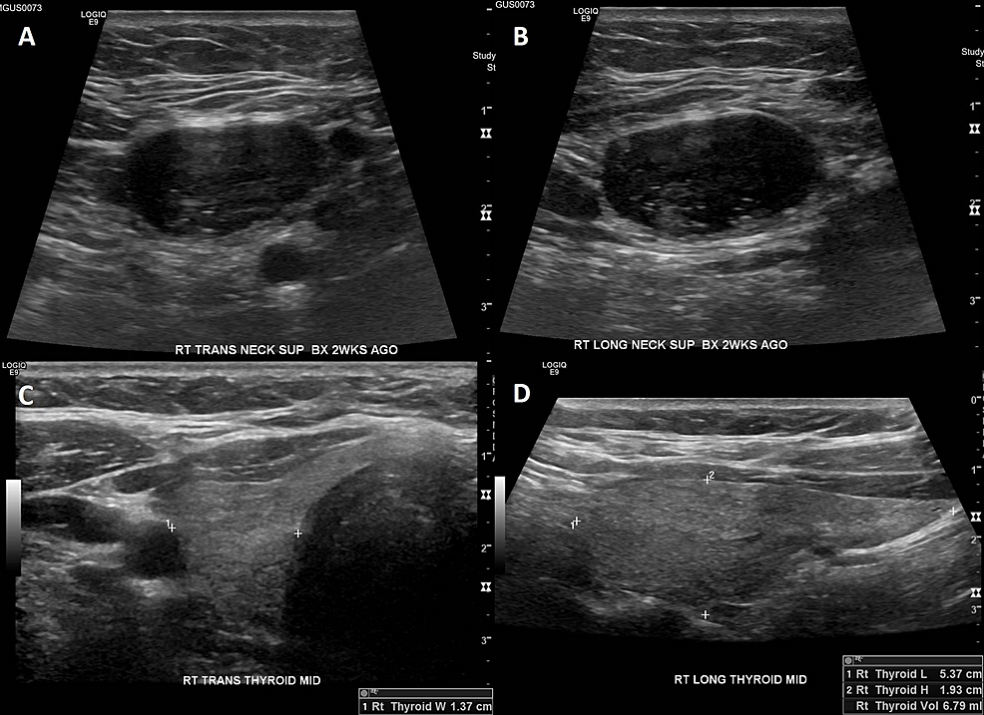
Ultrasound of right neck mass (A) Axial and (B) sagittal ultrasound images of the hypoechoic right neck nodule (level IIa) measuring 2.0 x 1.2 x 2.4 cm. (C) Transverse and (D) sagittal ultrasound images of the right thyroid lobe.
